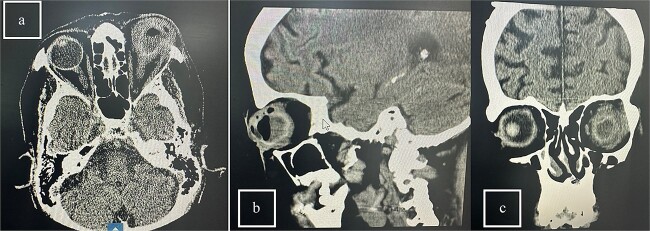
CT scans of the orbit for patient 5 showing (a) coronal view with dense fluid content and air-fluid leveling, (b) axial view with edematous changes in the intra-conal fat, and (c) sagittal view with involvement of the extra-ocular muscles.
